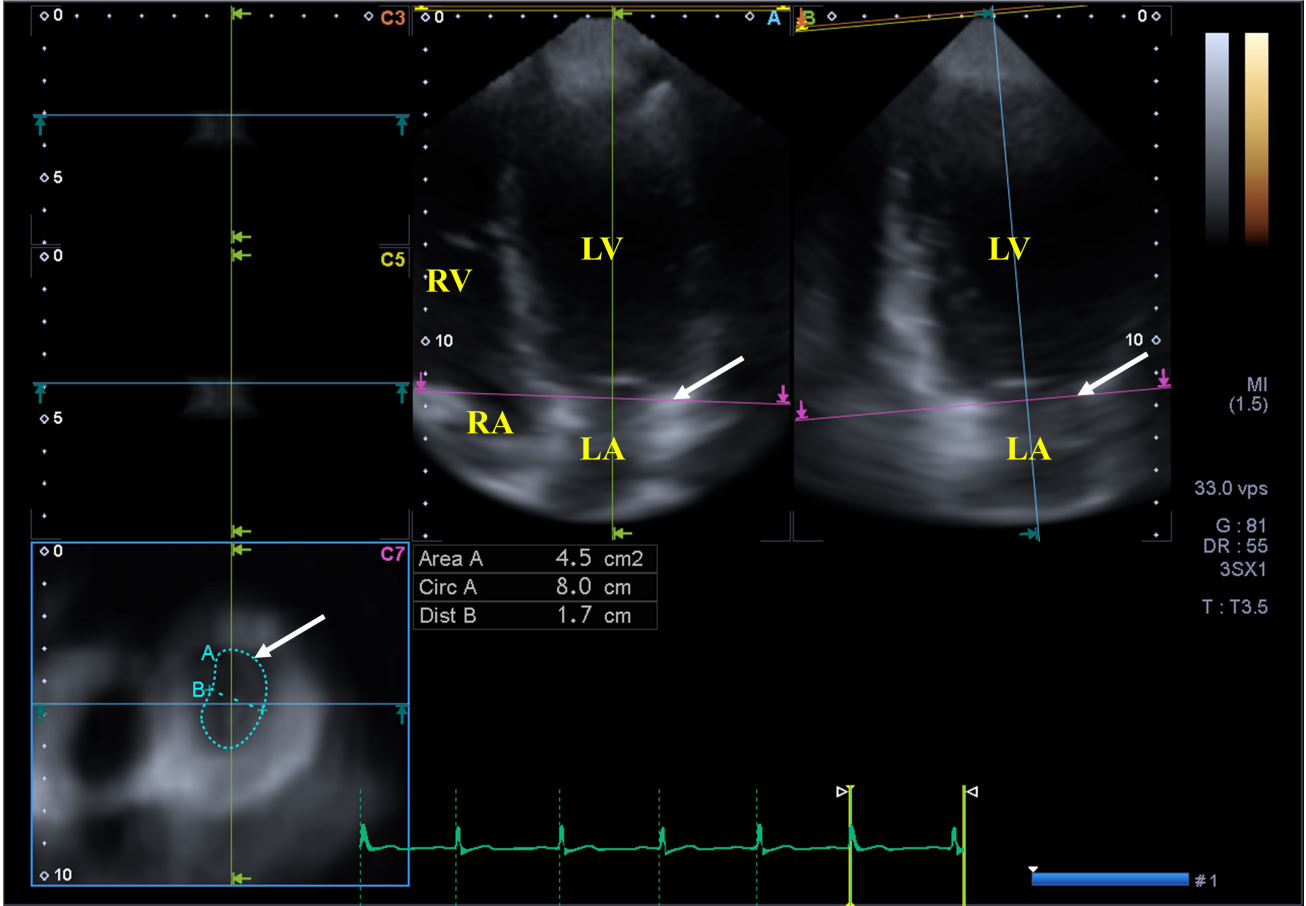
Three-dimensional speckle tracking echocardiography-derived
